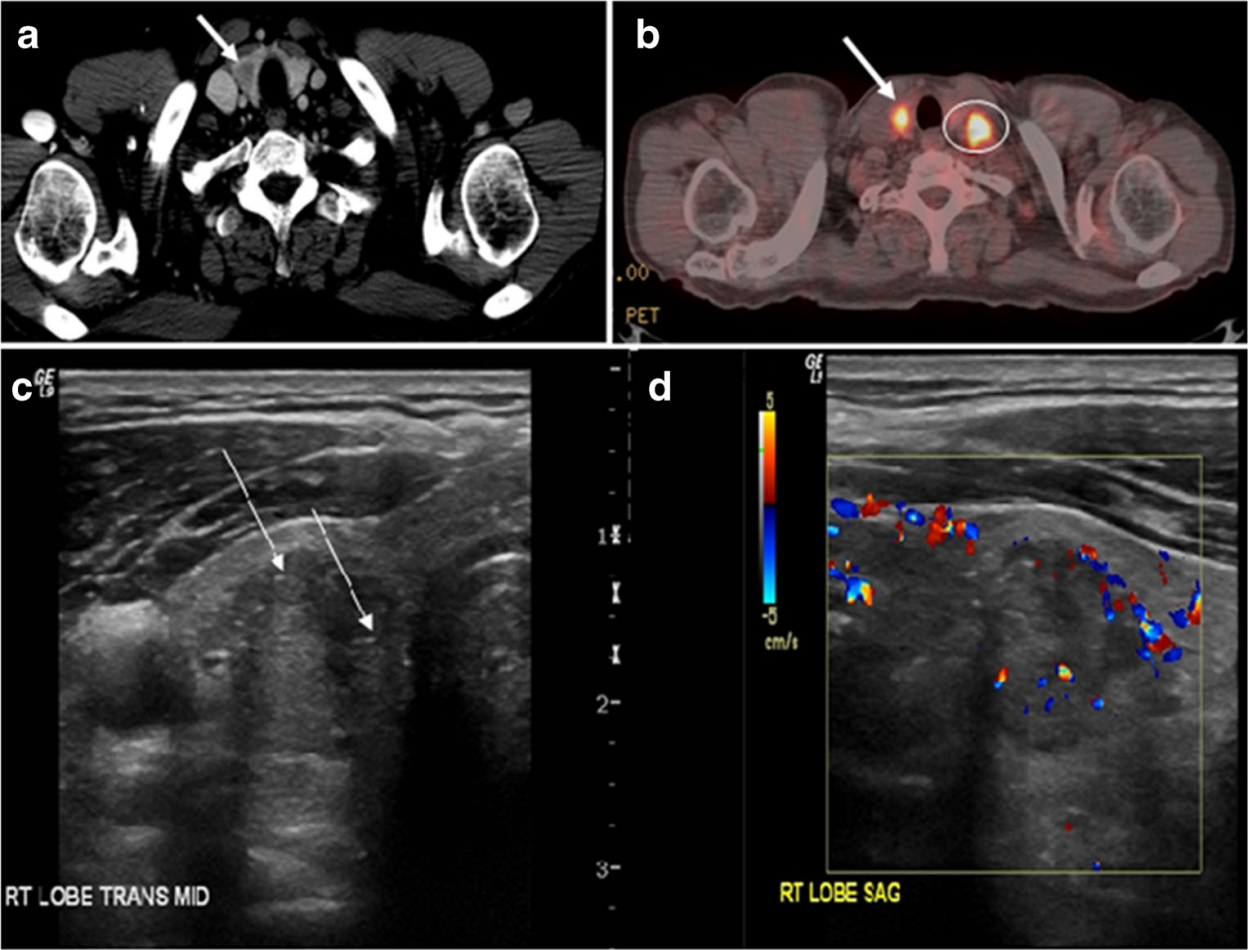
An incidental PTC in a 62-year-old male patient with lymphoma. a, b Enhanced axial CT scan and fused PET/CT scan of the neck demonstrate a well-defined, hypodense right thyroid nodule (*white arrow*) with high FDG uptake. The FDG-avid uptake in the left side (*circle*) is related to patient’s known lymphoma, which resolved after treatment. c, d Transverse greyscale and sagittal colour Doppler ultrasound of the neck demonstrate a right thyroid irregular hypoechoic lesion with some micro-calcifications (*white arrows*) and increased vascularity
